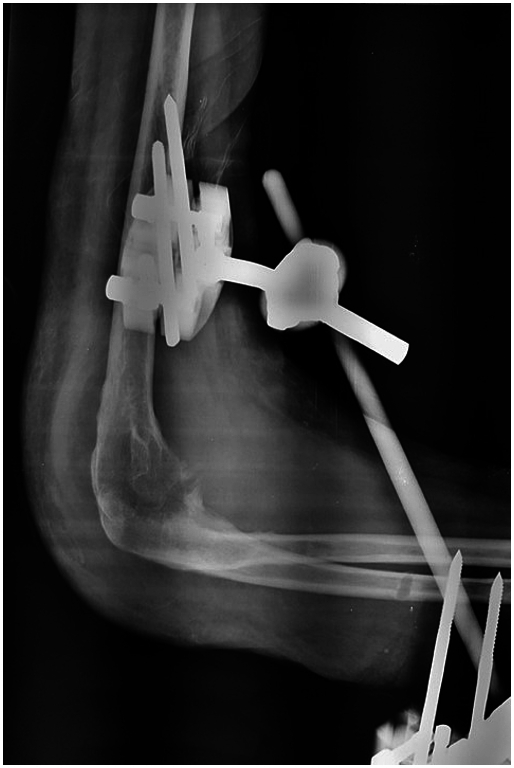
Lateral radiograph of the right elbow demonstrating successful stabilization achieved with an external fixator.
